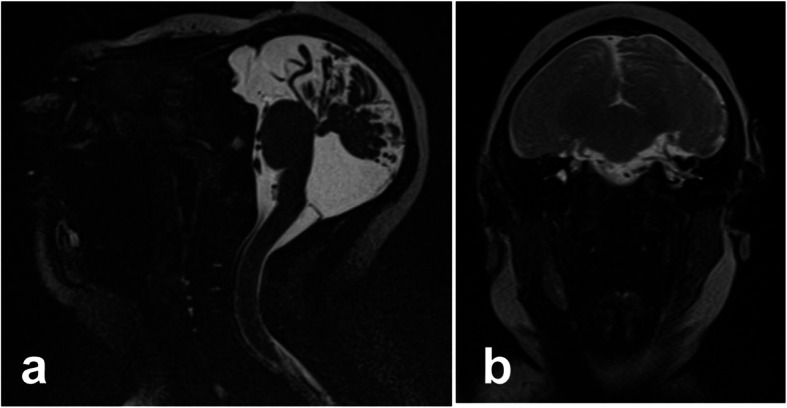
Magnetic resonance imaging (MRI) findings of the infant’s head. a T2-weighted sagittal image. b T2-weighted coronal image. On day 32 after birth, an MRI scan showed the absence of a cerebrum and a membranous structure in the region of the cerebrum with hydrocephalus
